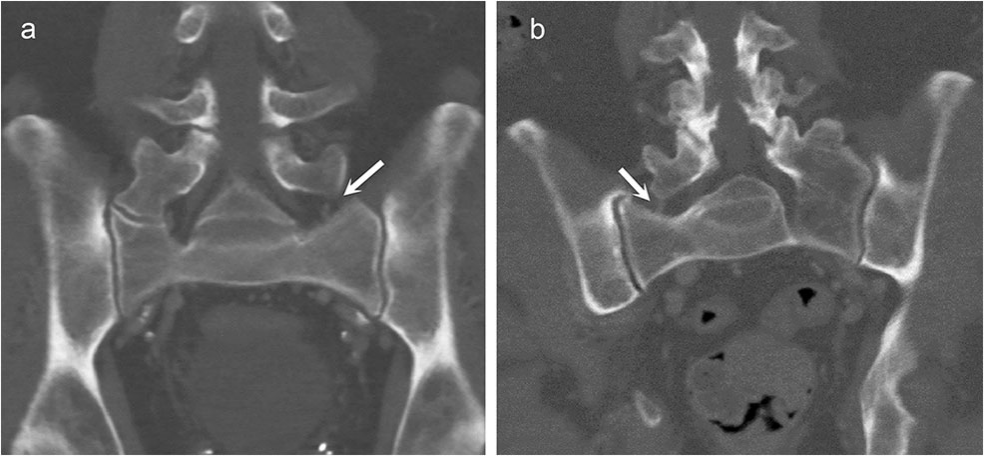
The proposed new subtypes to the classic Castellvi classification on coronal CT images. A 63-year-old male with suggested type IIc lumbosacral transitional vertebra (LSTV) showing a pseudoarticulation on the right side and an enlarged transverse process without articulation on the left side (arrow) (a). A 78-year-old male with suggested type IIIc LSTV demonstrating a complete fusion on the left side and an enlarged transverse process without articulation on the right side (arrow) (b)
